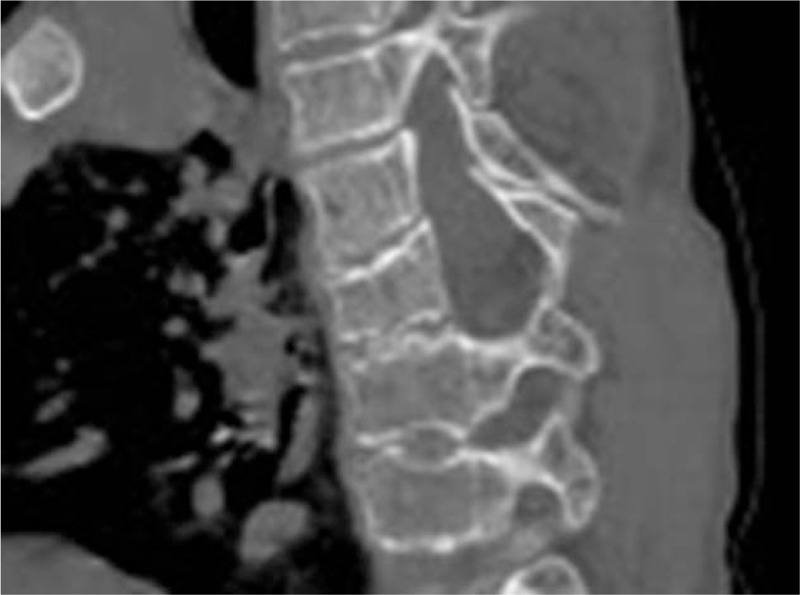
Coronal 3D-CT scan of the thoracic spine of the proband at age of 12-year showed wedging of 3 consecutive adjacent vertebral bodies in the apex of the kyphosis, irregular vertebral apophyseal lines combined with flattening and wedging, narrowing of the intervertebral disc spaces and variable presence of Schmorl's nodes, causing effectively the development of thoracic kyphosis (arrows). CT = computed tomography.
